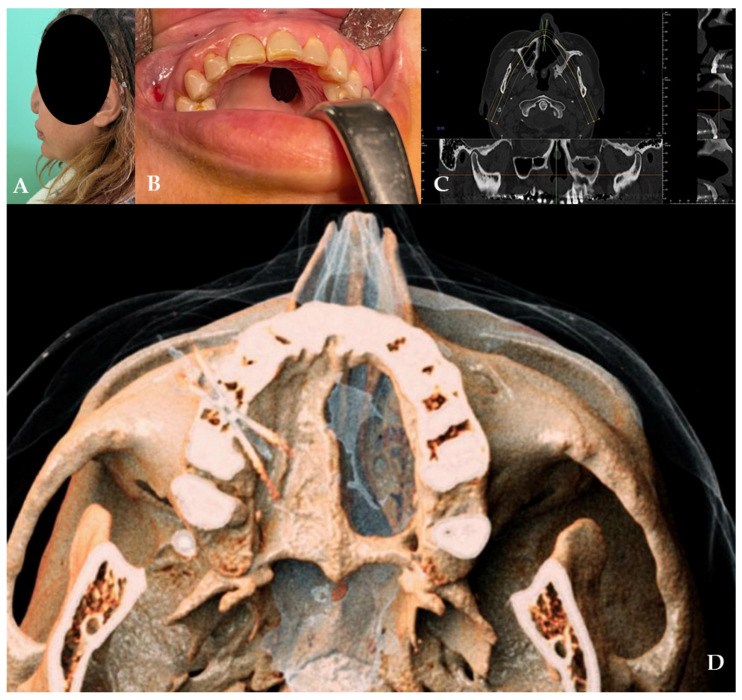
(A,B) Clinical presentation of the oro-nasal communication. In inset (A), it is possible to appreciate the collapse of the nasal pyramid. (C,D) Radiographic and 3D reconstruction using InVivo6^®^ and Anatomage Table EDU vers.8^®^ (Santa Clara, CA, USA) software. DICOM files were imported in InVivo6^®^ (Santa Clara, CA, USA) software to obtain the axial, cross, and panorex sections. Afterwards, the DICOM files were imported Anatomage Table EDU vers.8^®^ (Santa Clara, CA, USA) to obtain the 3D rendering.
